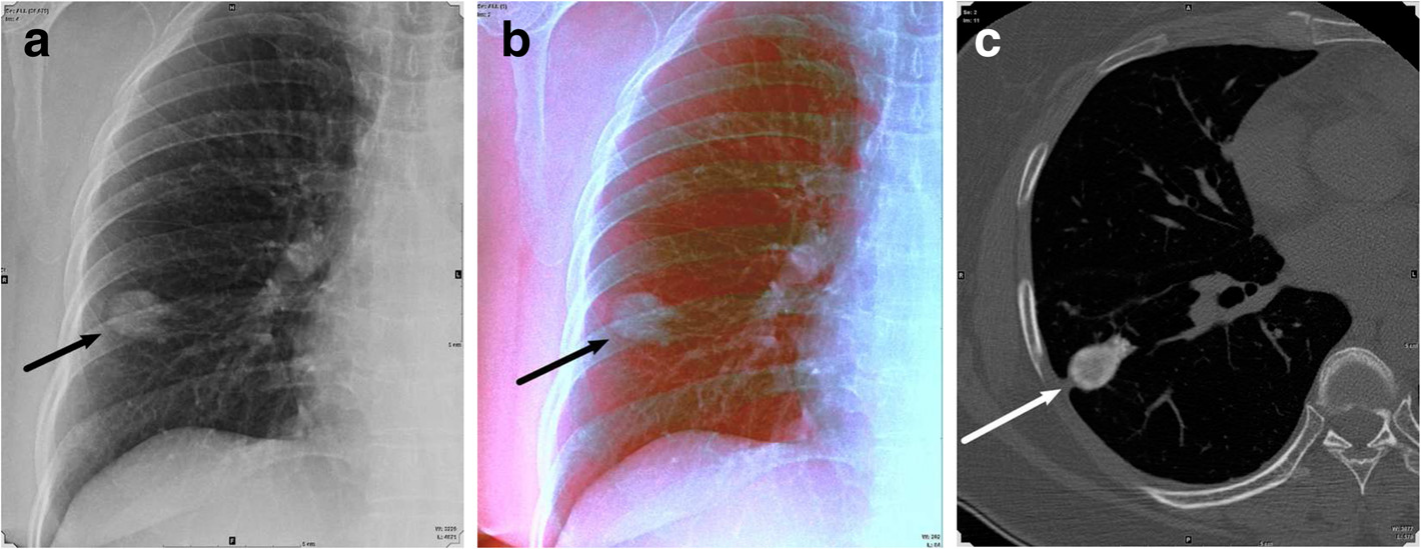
Conventional gray scale radiography (GSR) and dual-energy radiography with color-representation (DCR) of a 60-year-old woman. a Conventional GSR image shows a nodular opacity in right lower lung zone (*arrow*). b DCR image shows distinct blue color of the nodular opacity (*arrow*) indicating calcified nodule. c Axial CT image shows a calcified granuloma in the right lower lobe (*arrow*)
